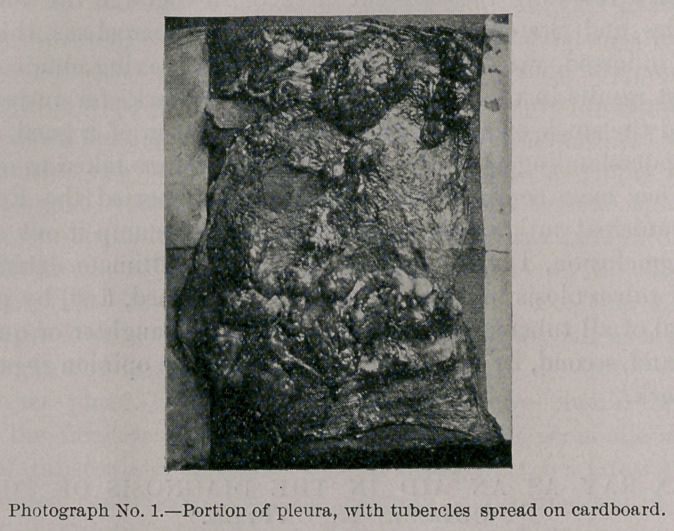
Radiograph No. 1. f2:
